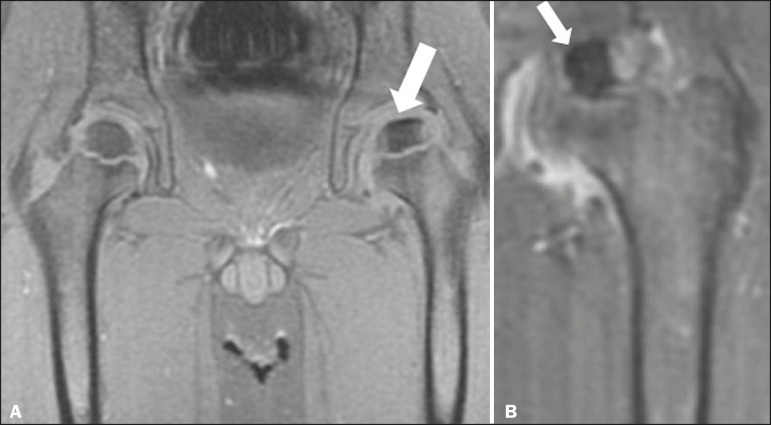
MRI of the same patient shown in Figure 1. A: Unenhanced
